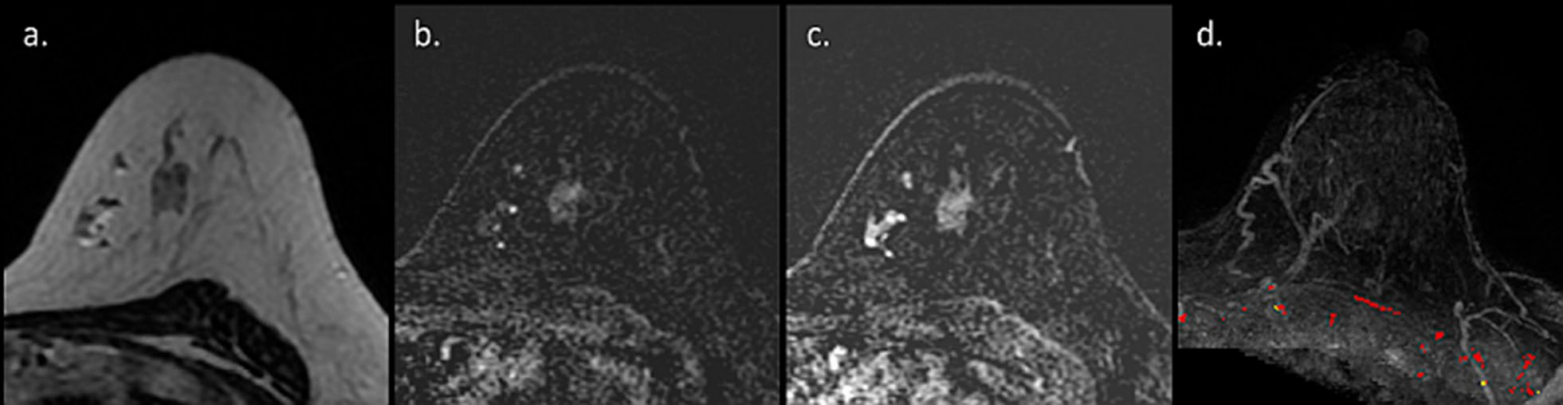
MRI of lactating adenoma. MR images of patient presented with palpable mass on the left breast 2 months after breastfeeding cessation are presented. T2 weighted image showed a hypo-intense mass (a), which exhibits a gradual enhancement and early and late DCE (b, c), below the signal intensity threshold for suspicious findings on CAD (d). Despite these benign features, the patient underwent vacuum-assisted biopsy which revealed lactating adenoma
